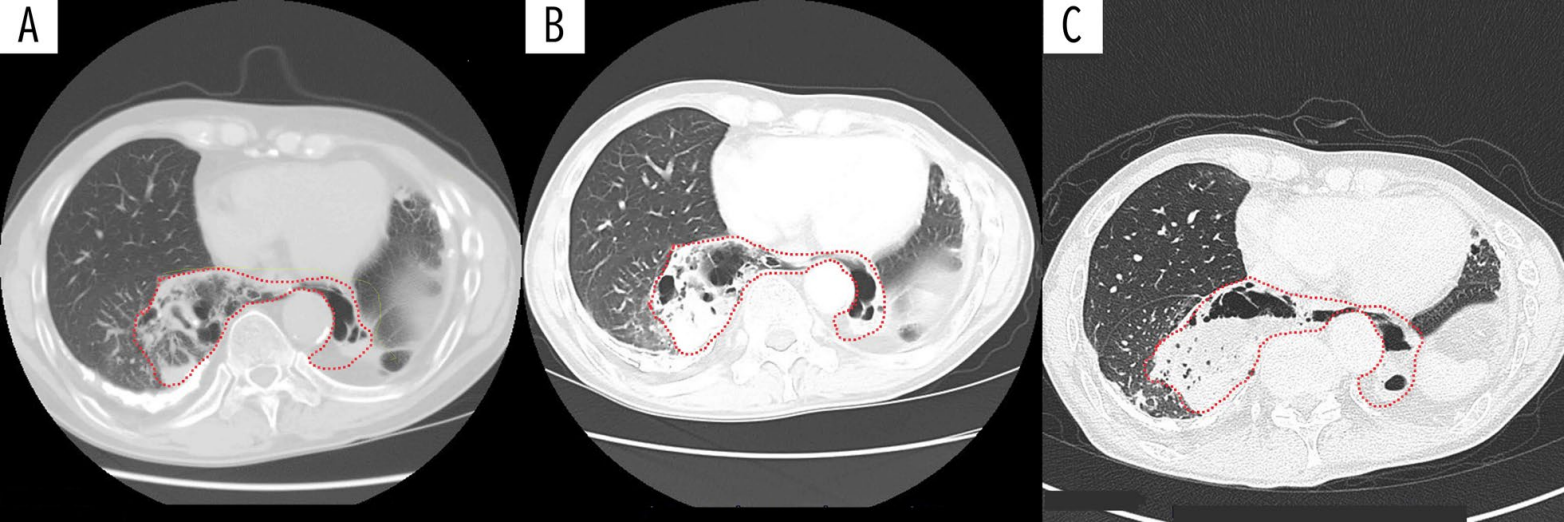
Computed tomography at 5 years (A), 3 years (B), and 1 year (C) before surgery. The horseshoe lung (dotted line) became rough because of repeated infections
